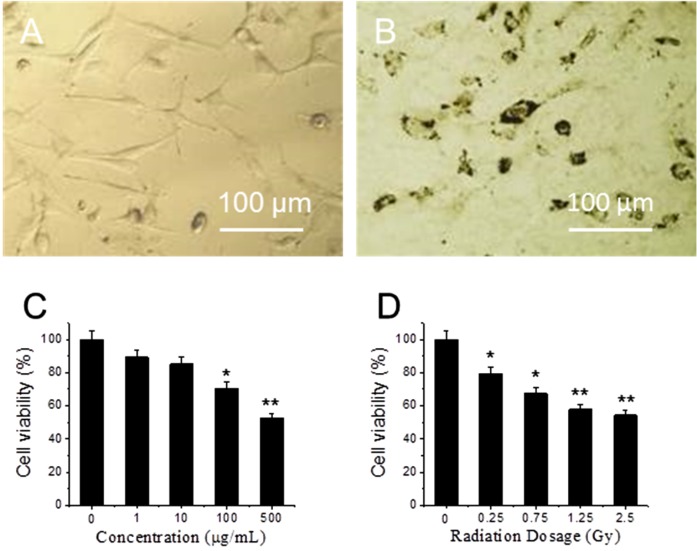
Optical images of cells (A) and cells treated with graphene oxide (GO) for 24 h (B); cytotoxicity of cells treated with different concentrations of GO (C); and exposed to different dose of X-ray (D). “*” (*p* < 0.05) and “” (*p* < 0.01) represent significant difference and extra significant difference, respectively.
